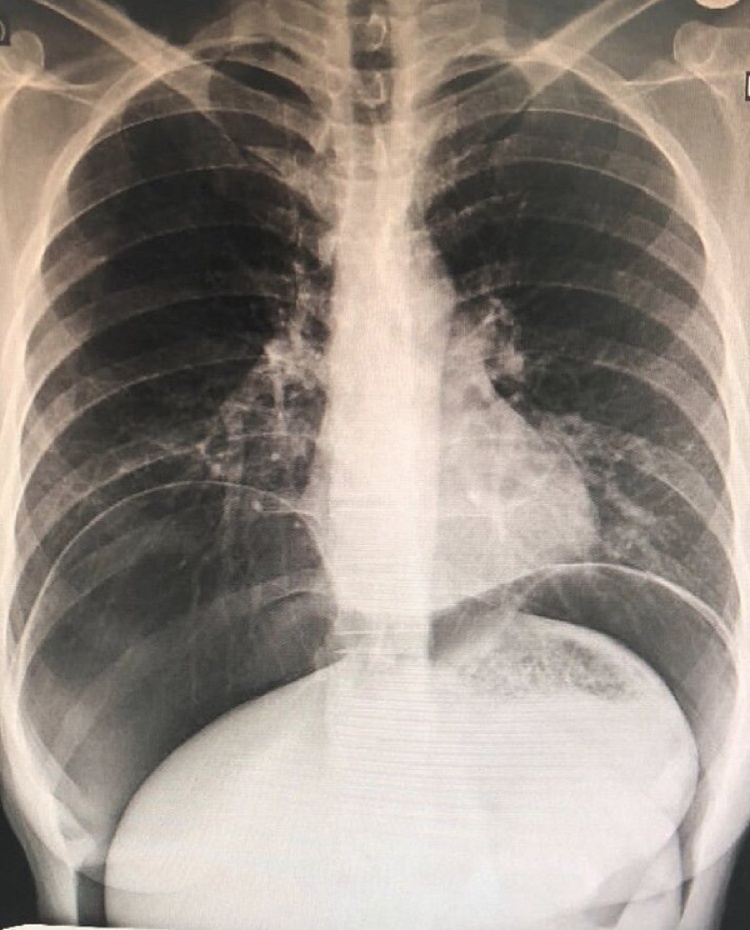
Chest x-ray showed significant bilateral pneumoperitoneum with notice of the gastric band.
